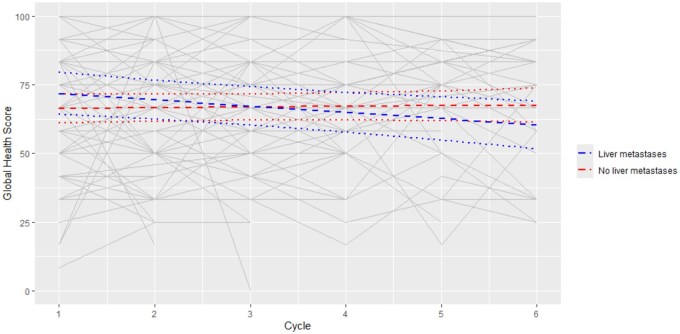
Global Health Status (GHS) during EP-CT participation stratified by liver metastases. Solid lines represent mean predicted GHS scores over cycles, with separate lines for patients with liver metastases and without liver metastases. Shaded bands/dashed lines represent 95% confidence intervals. Baseline median GHS for all participants was 67/100. This score is derived using the EORTC-QLQ-C30 Scoring Manual which converts the raw score out of 4 to a toxicity score out of 100. While there was no significant difference in the GHS between patients with and without liver metastases at any individual cycle, patients with liver metastases had a significant reduction of 2.29 in GHS per cycle (95% CI = −3.9 to −0.69, *P* = .0054).
